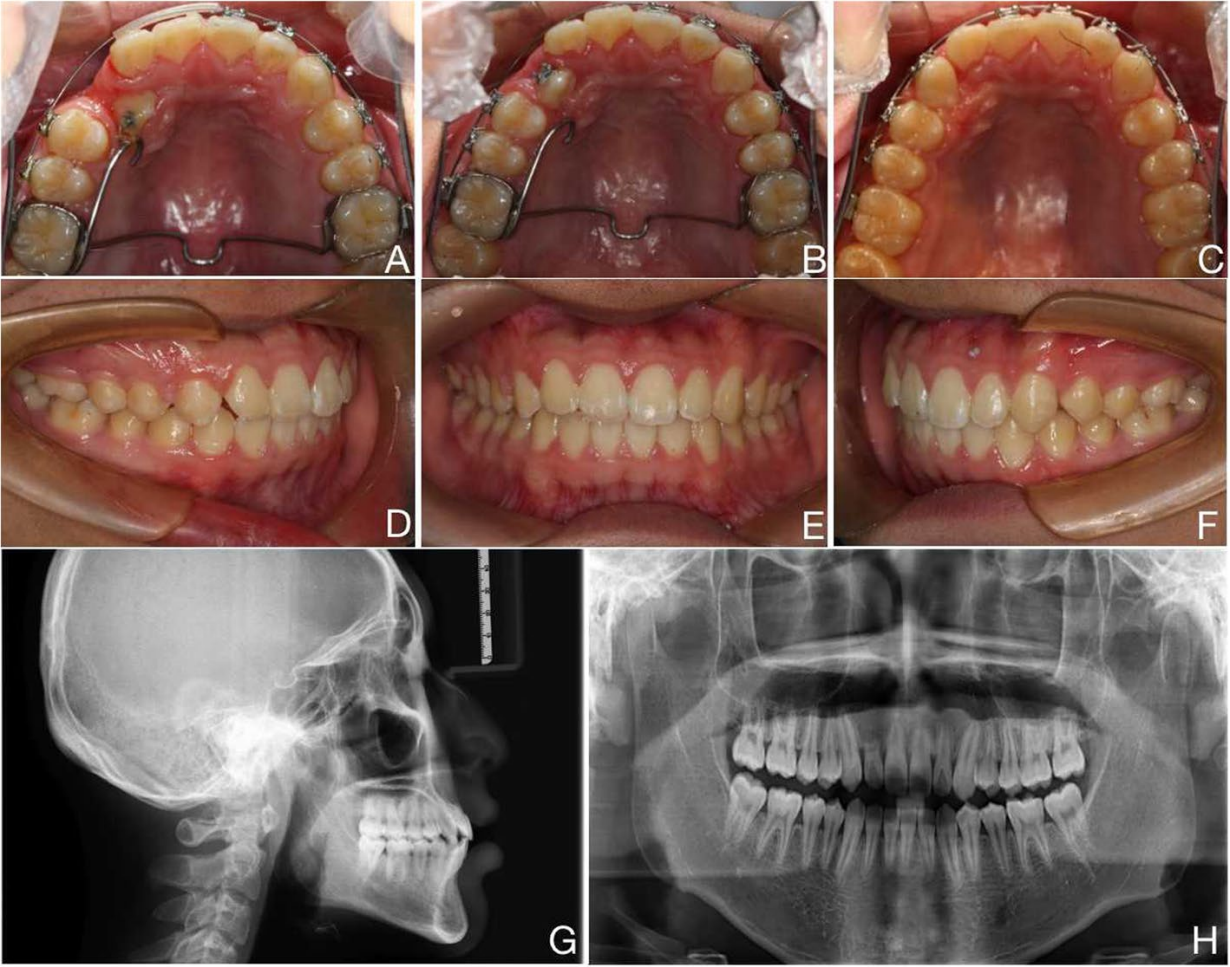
Intraoral images during treatment, intraoral images, and radiographs after treatment
